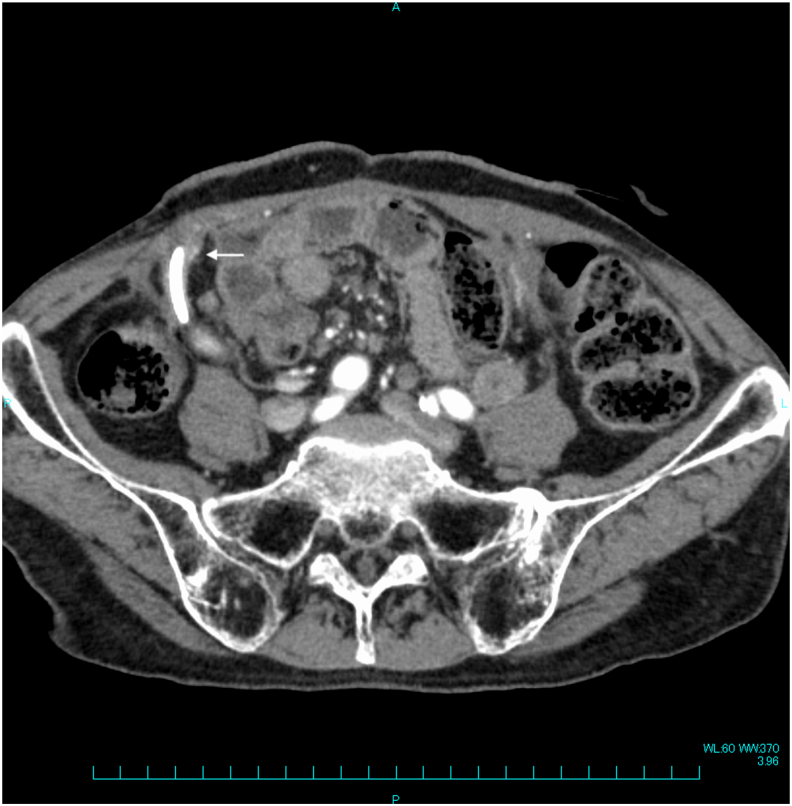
Contrast-enhanced computed tomography revealed an unnatural dilated right inferior epigastric artery located near his urethral catheter (arrow).
